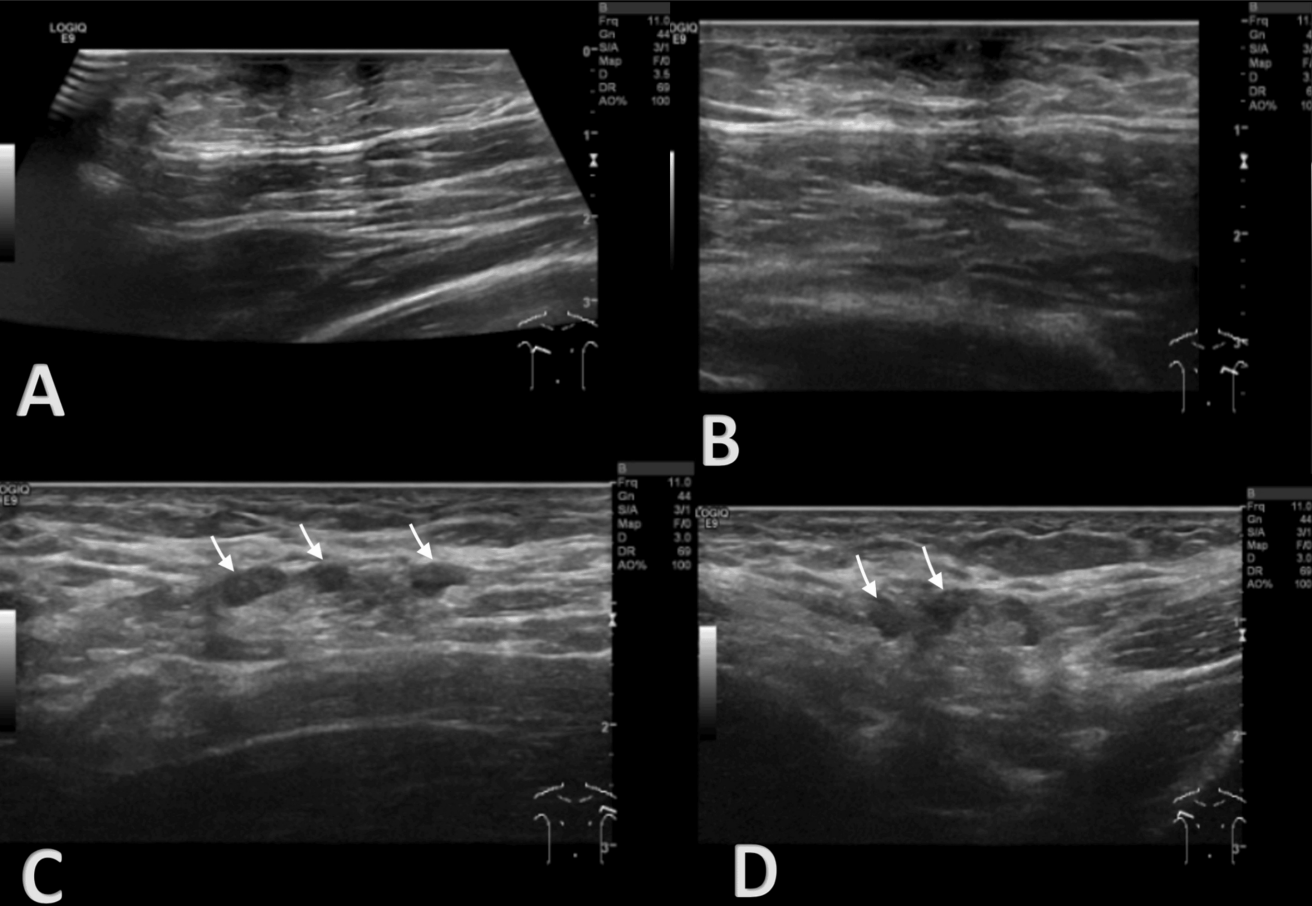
Breast ultrasound A: right breast; B: left breast; C: left axilla; D: right axilla Findings show mild bilateral dendritic gynecomastia (A, B) and bilateral reactive axillary lymphadenopathy with preserved fatty hilum (white arrows in C and D).
